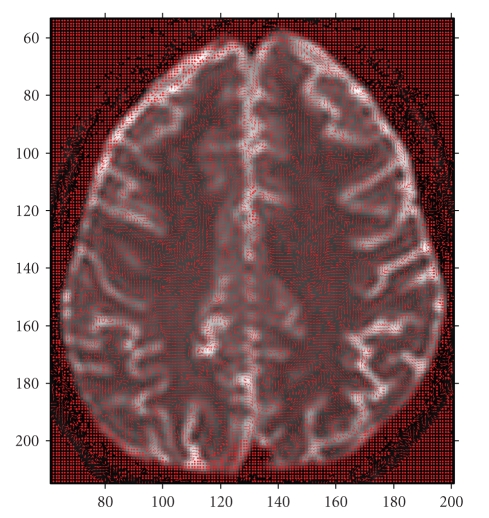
Calculated principal eigenvectors of the entire slice superimposed on axial brain MR image.
